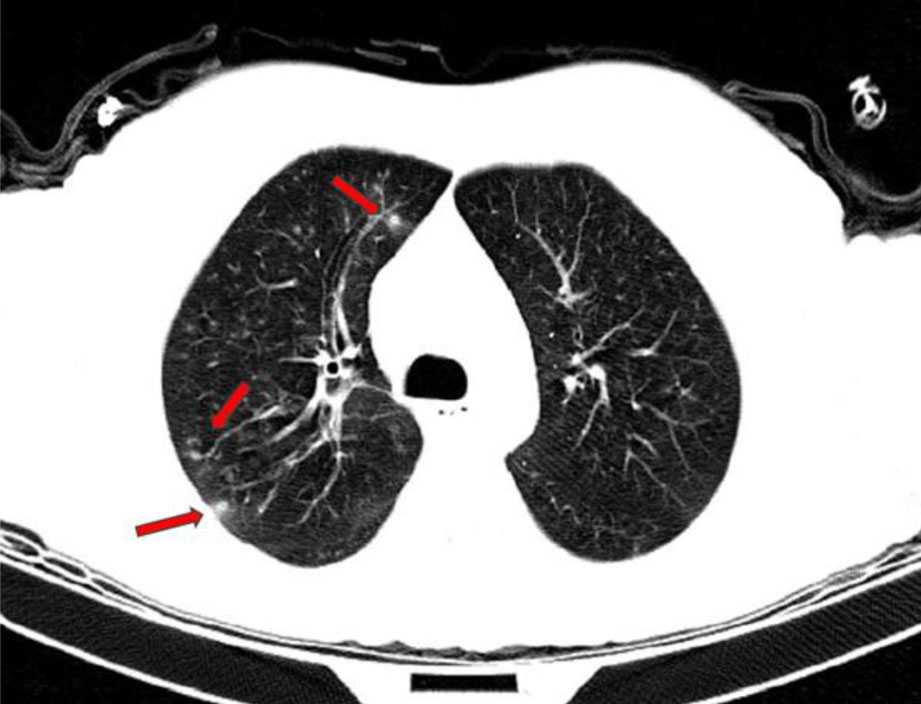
Lung computed tomography scan showing multiple nodules in the right upper lobe of the lung (head of the red arrows)
